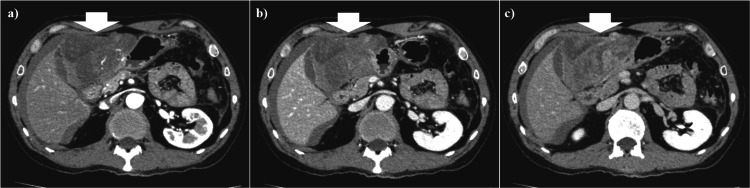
Abdominal computed tomography (CT) on admission showing an intra-abdominal peri-hepatic fluid collection with high density, which could suggest hemoperitoneum. (a) Early phase. (b) Portal phase. (c) Delayed phase. Contrast-enhanced abdominal CT shows a large heterogeneous mass with a non-uniform enhancement pattern (white arrow) at the extraluminal space of the gastric anterior wall and intra-abdominal peri-hepatic fluid collection.
